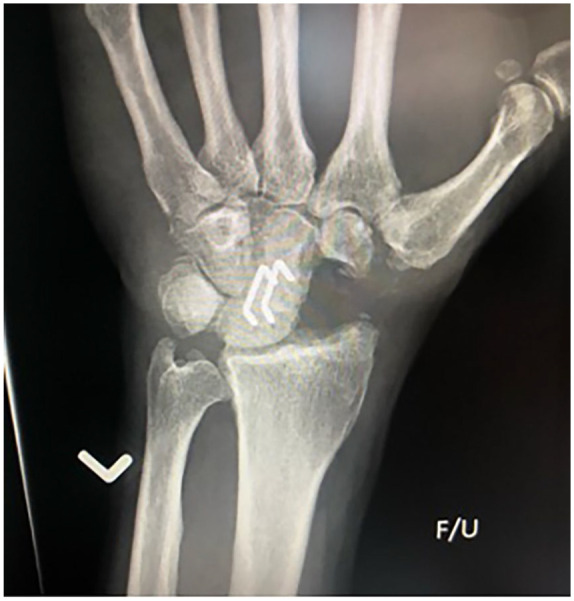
Anteroposterior radiograph of a 2-corner fusion using staples between the lunate and capitate. The scaphoid has been excised.
